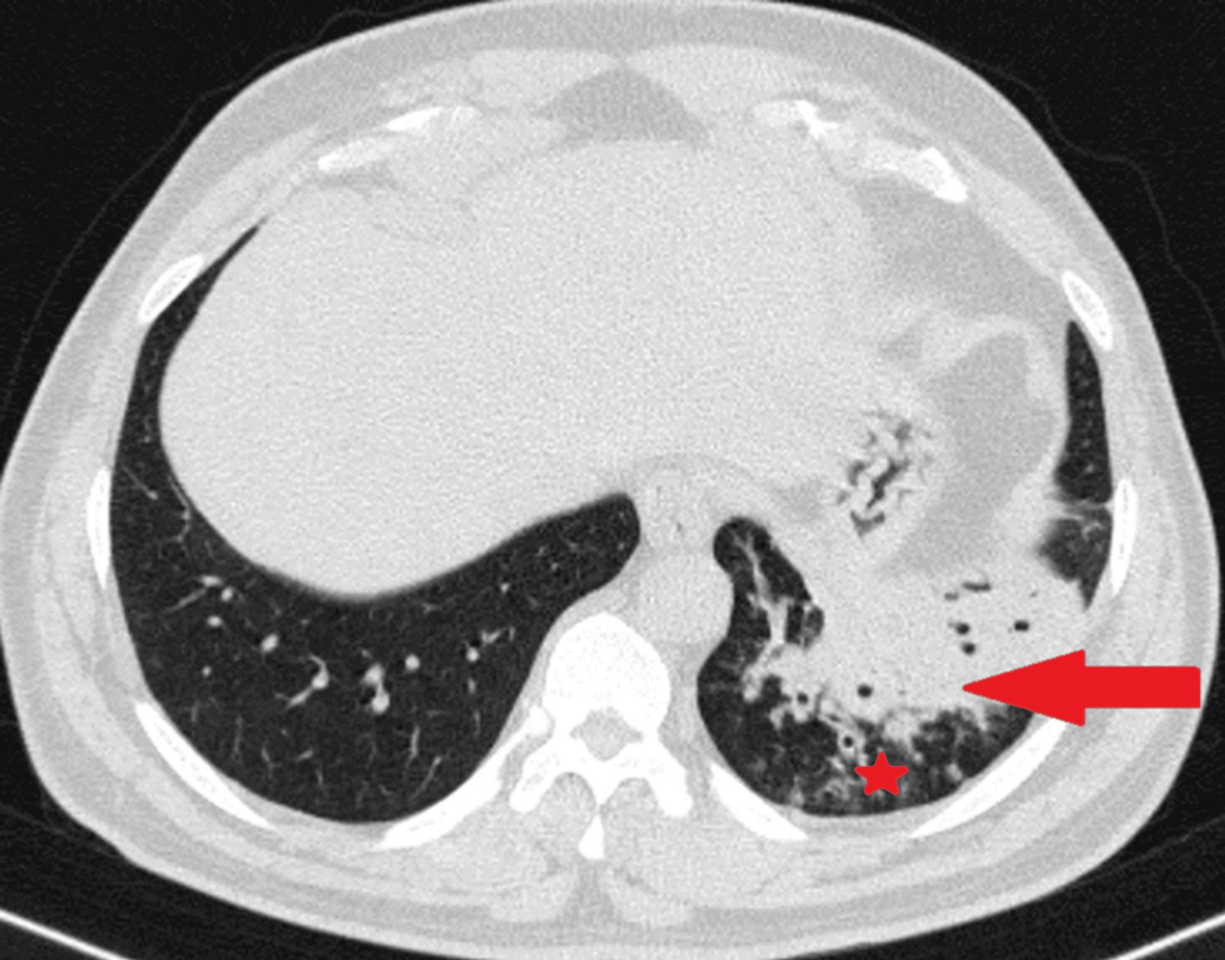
High-resolution computed tomography of the chest lung window axial section showed consolidation in the left lower lobe segments (red arrow) and a tree-in-bud appearance (star), representing aspiration pneumonitis.
